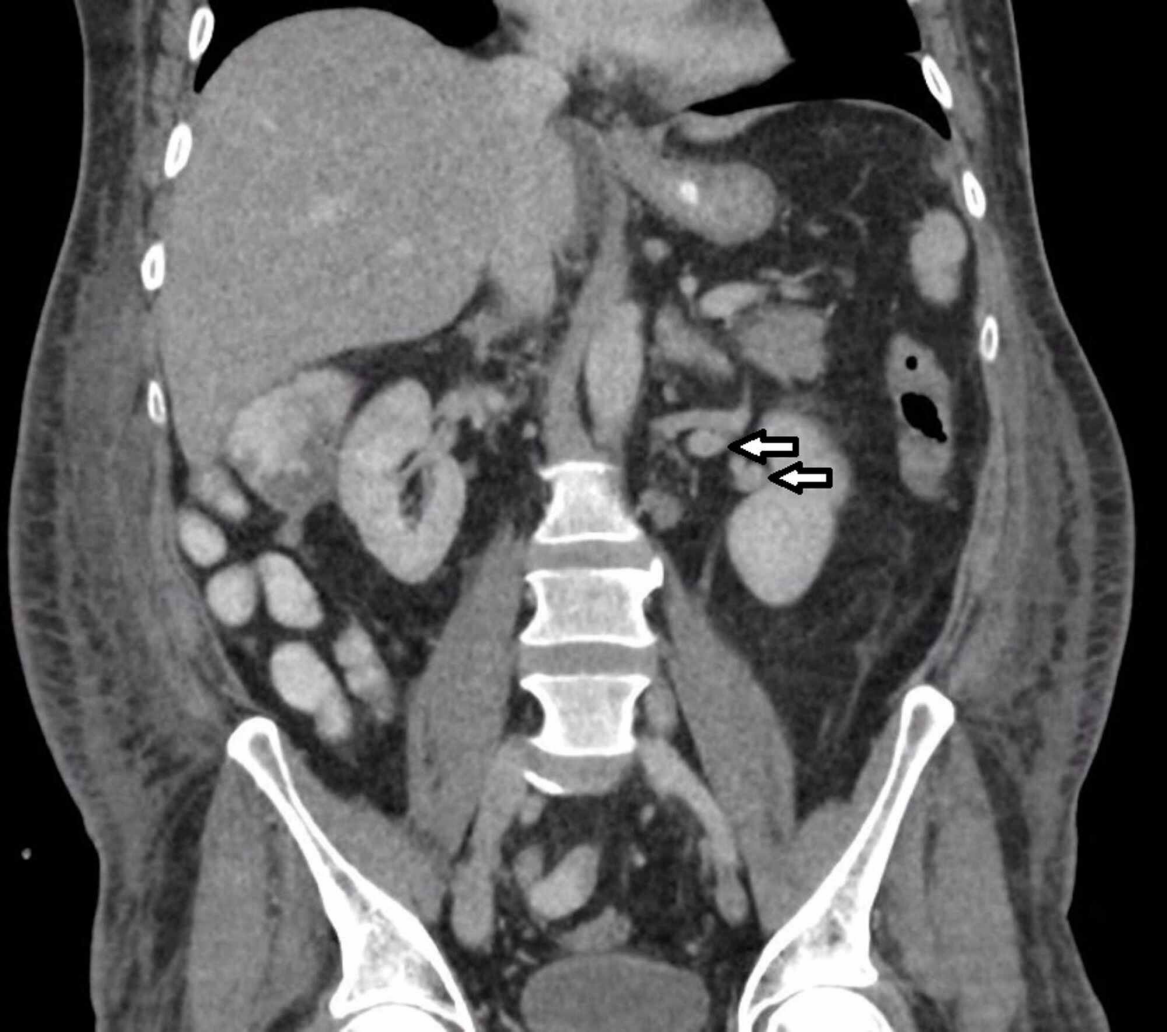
CT abdomen (coronal): Superior and inferior renal veins leaving the left kidney.
